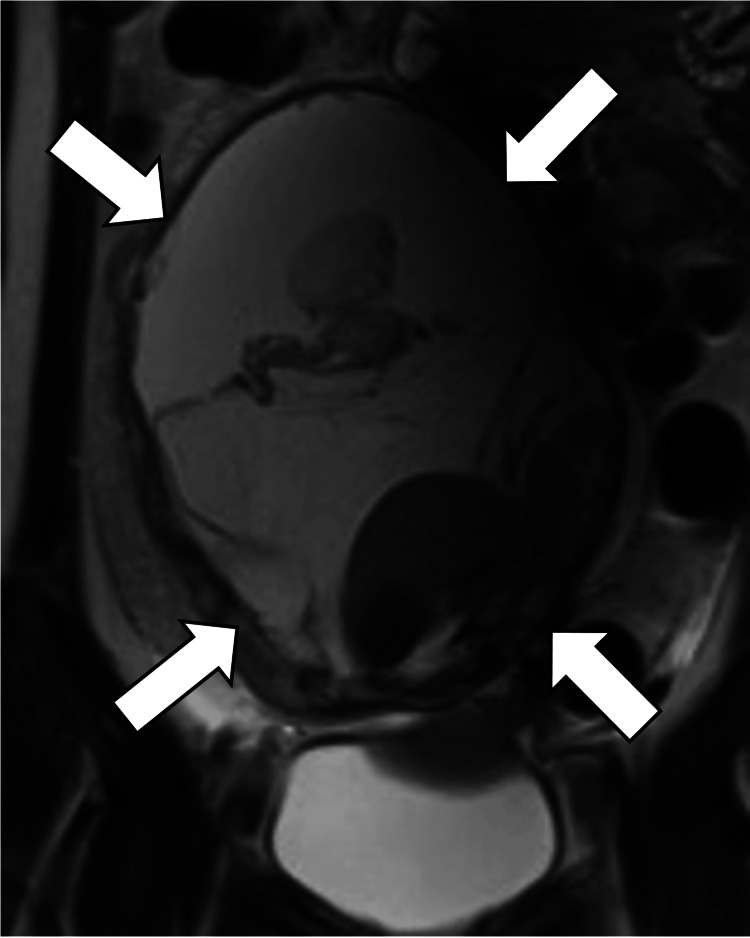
Coronal, T2-weighted T2-weighted image shows a voluminous multilocular cystic mass (arrows) with several septations.
